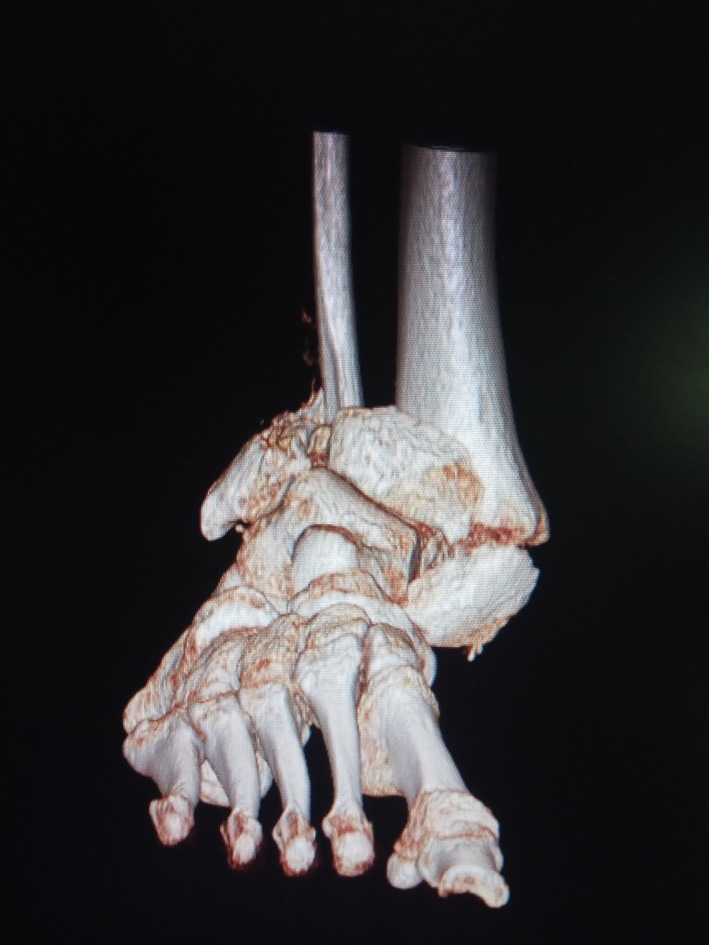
CT reconstruction of Charcot joint
